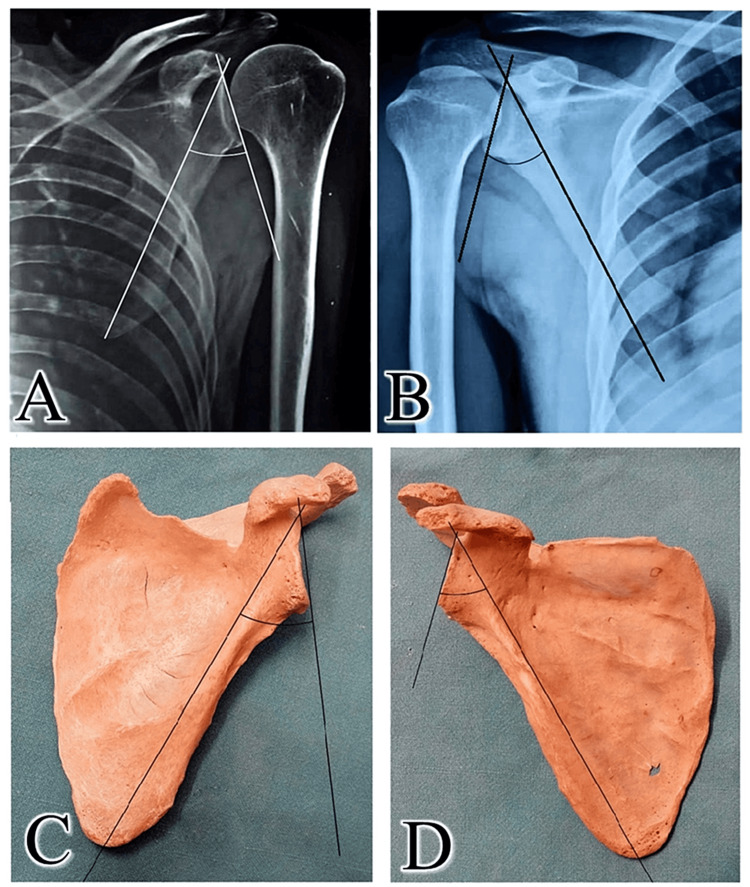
The GPA angle of the scapula acquired by various methods. A) left scapula (Neer I view) in radiograph of the shoulder region; (B) right scapula (AP view) in the radiograph of the shoulder region; (C) ventral aspect of left scapula; and (D) ventral aspect of right scapula. GPA: glenopolar angle; AP: anteroposterior; Neer’s I view: Neer method is an X-ray examination of the shoulder using tangential projection of central ray at 10 to 15° angulation.
